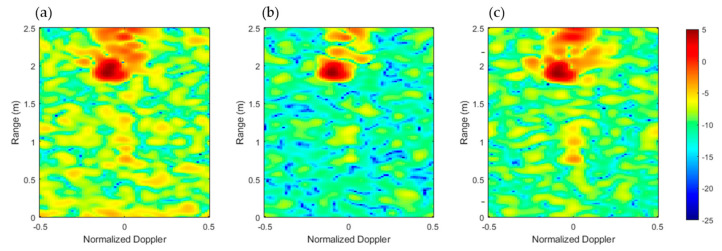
Range–Doppler (dB) frame for antenna no. 1 (a), antenna no. 2 (b), and antenna no. 3 (c), related to WALK activity.
